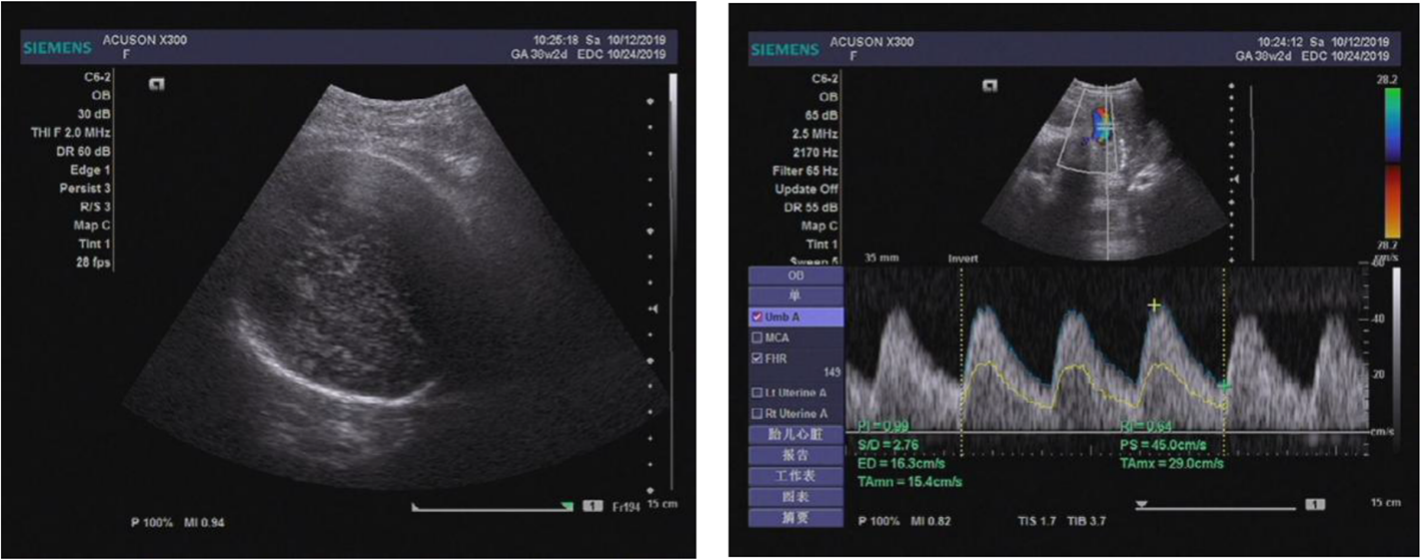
Antenatal ultrasound scan at 36 + 5 weeks gestation, and an ultrasound scan detected an echo-free cyst (5.0 cm × 5.4 cm × 6.8 cm) with a head circumference of 32.1 cm and a CVR of 2.99
